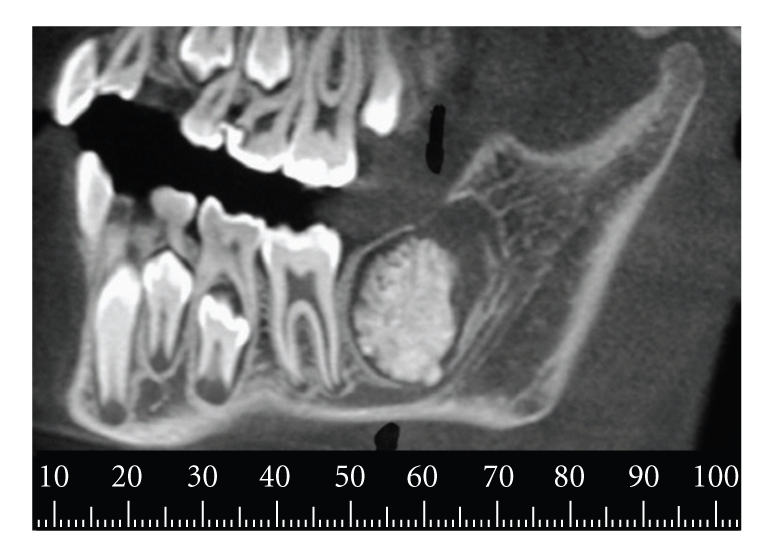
Cone-beam tomography (panoramic reconstruction) showing a well-circumscribed calcified mass in intimate contact with the alveolar inferior nerve.
